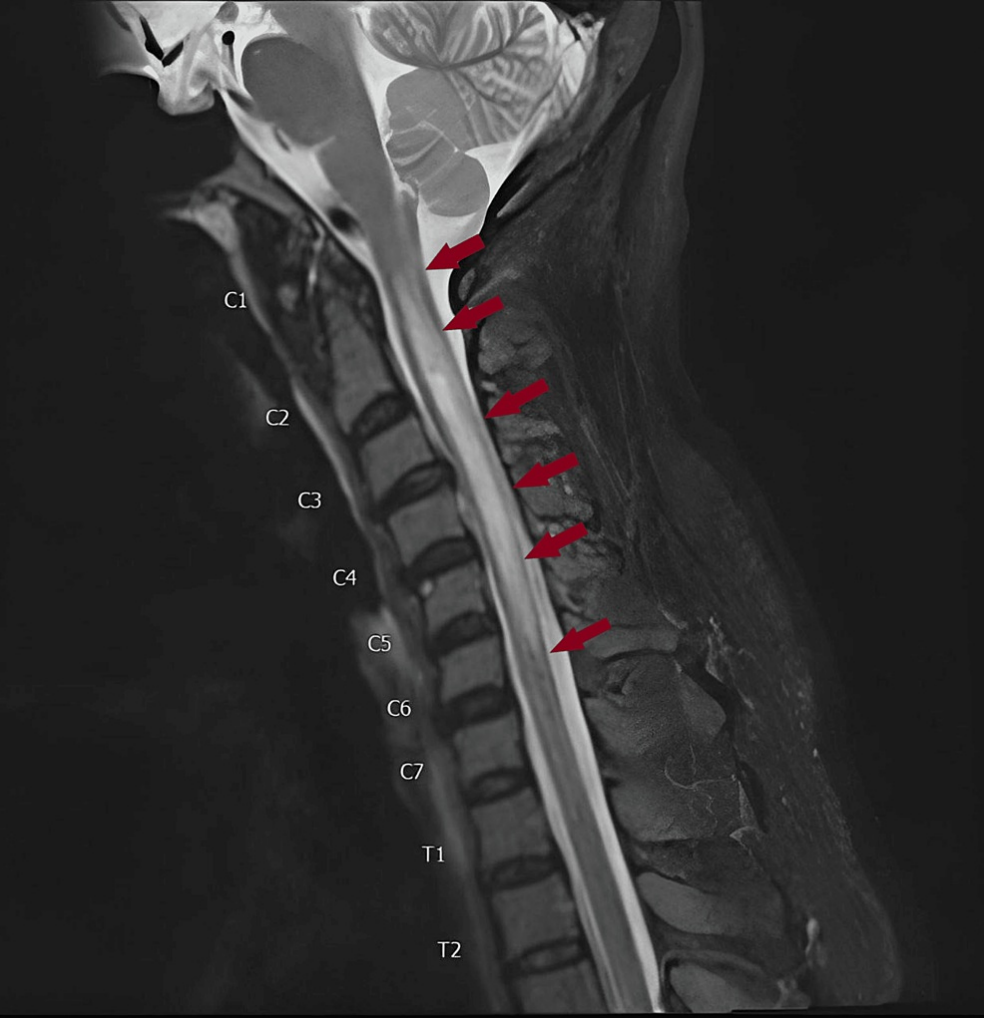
Sagittal T2-weighted image of the cervical spinal cord MRI demonstrating a focus of enhancement from C1 to C4, measuring 8 x 7 x 45 mm with ill-defined margins. Extensive edema extending from C1 to mid-C6 vertebra can also be appreciated (red arrows).
